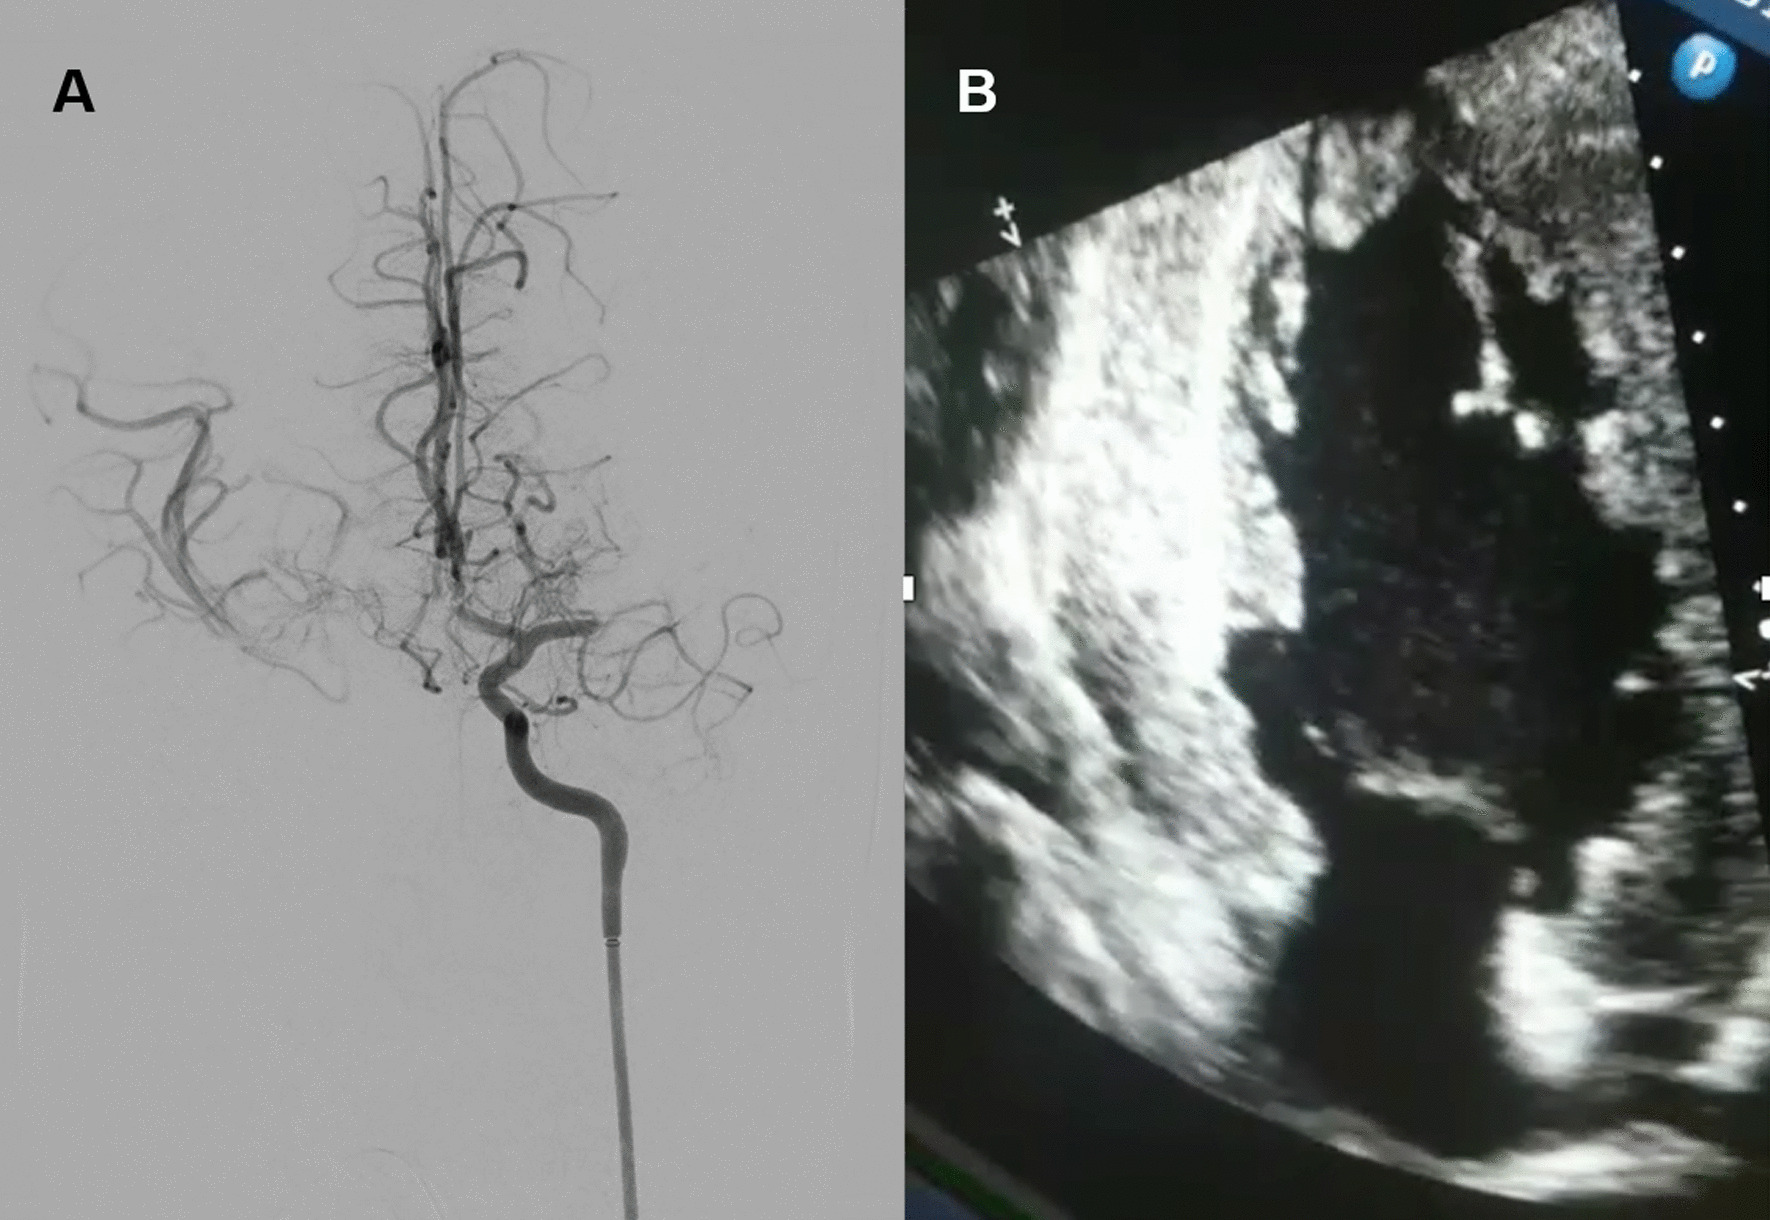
A Cerebral angiography during procedure demonstrating a complete occlusion of the left middle cerebral artery bifurcation. B Echocardiogram revealed a hypertrophic cardiomyopathy and an echogenic mass at the left ventricular apex suggestive of intracardiac thrombus
